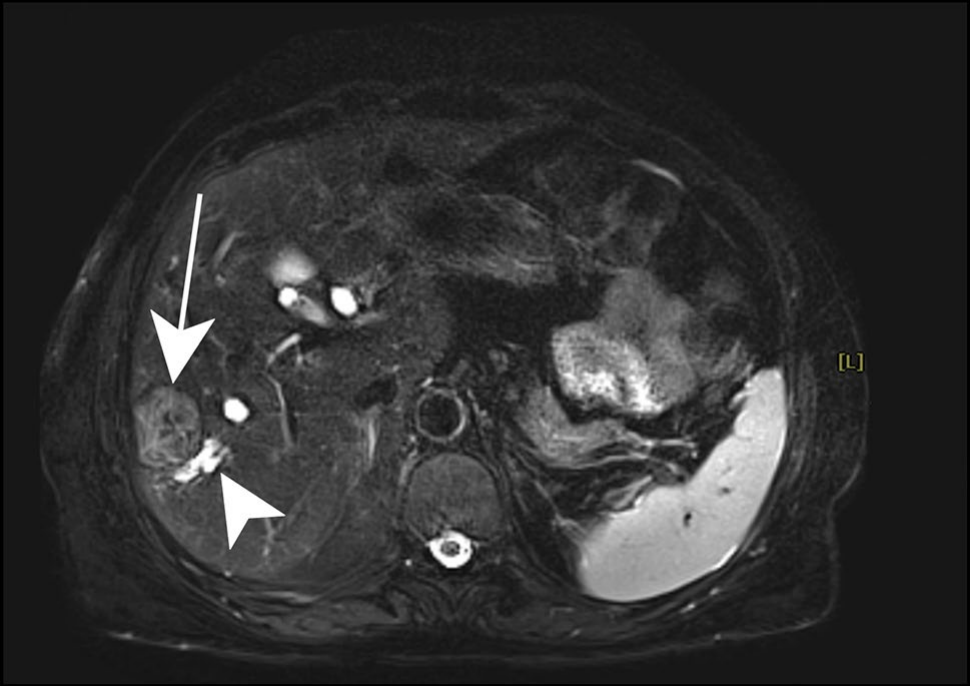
Cystic echinococcosis type CE4 in a 62-year-old female patient with upper abdominal pain. Axial fat-suppressed T2-weighted image shows a well-defined subcapsular moderately hyperintense lesion (white arrow) with a characteristic “ball of wool” sign. An adjacent dilated intrahepatic bile duct is also noted (white arrowhead)
